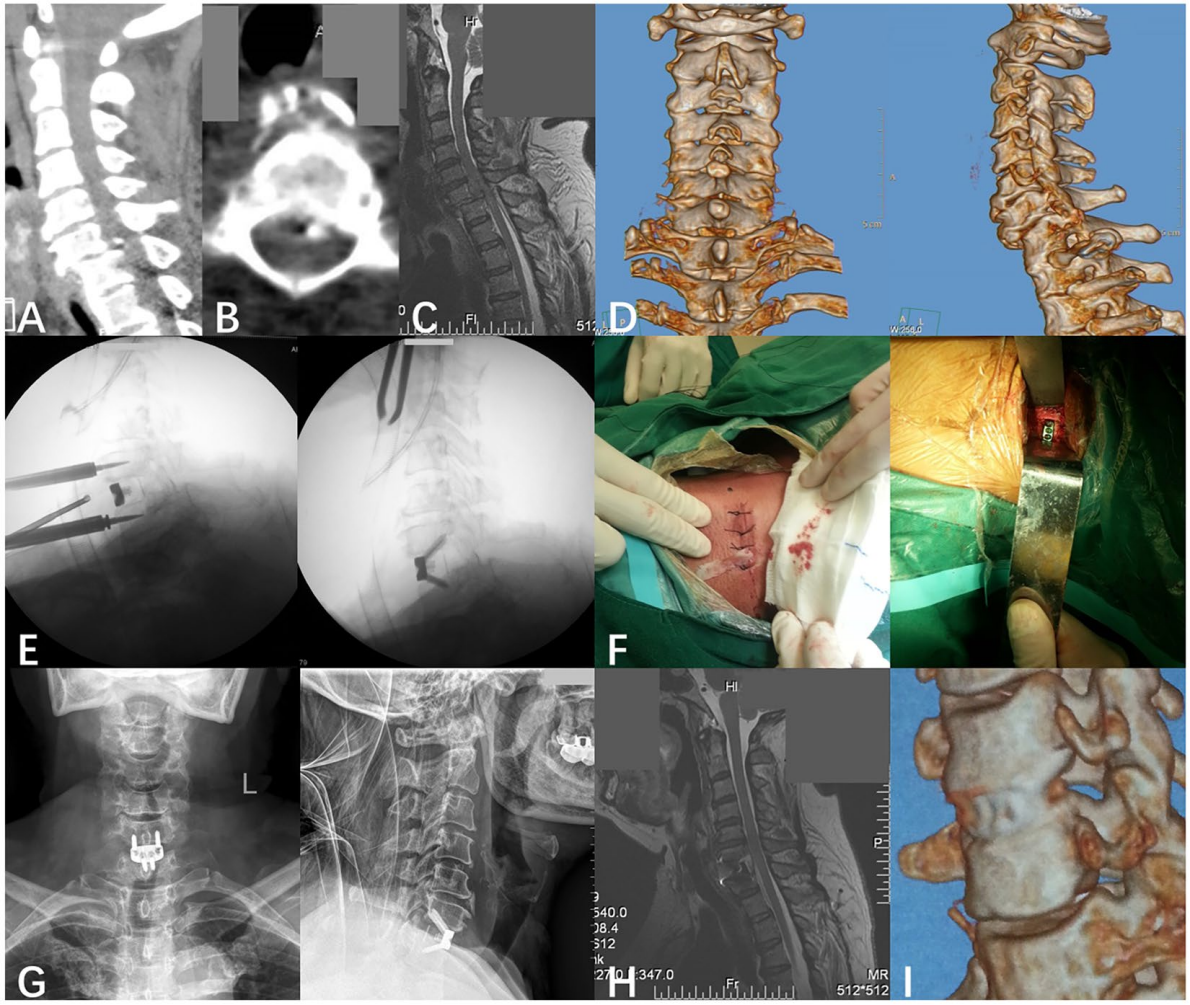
Protocol patient with C6/7 dislocation and right-side facet interlocking. (A) At presentation, sagittal position CT indicates C6/7 dislocation, and a small bone fragment presents at the posterior margin of the vertebral body of C6. (B) Horizontal position CT reveals a small bone fragment presents at the posterior margin of the vertebral body of C6. (C) Sagittal position MRI reveals C6/7 dislocation, disc herniation and compression of the spinal cord. (D) Stereoscopic reconstructed CT reveals C6/7 dislocation and facet interlocking at the right-side of C6. (E) Intraoperative C-arm fluoroscopic visualization. (F) Operative incision. (G) The anteroposterior and lateral view of cervical spine radiography after surgery. (H) Two weeks after the surgery, the MRI indicates the cervical sequence and stability was reconstructed, and the compression of the spinal cord was released completely. (I) Three months after the surgery, the reconstructed CT reveals complete postoperative realignment and fusion was achieved.
